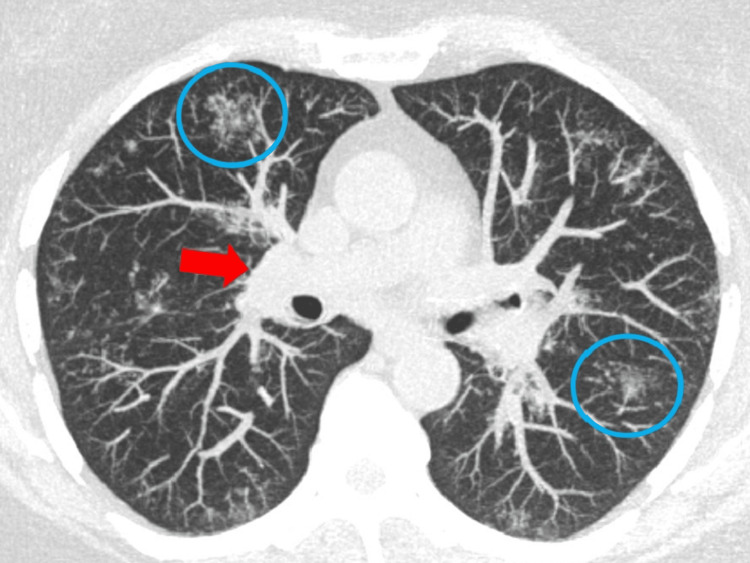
Chest CT image. Axial MIP non-contrast chest CT image showed perilymphatic micronodules in both lungs (larger clusters demarcated with blue circles) and hilar lymphadenopathy (red arrow). These findings are consistent with sarcoidosis. MIP, maximum intensity projection; CT, computed tomography
